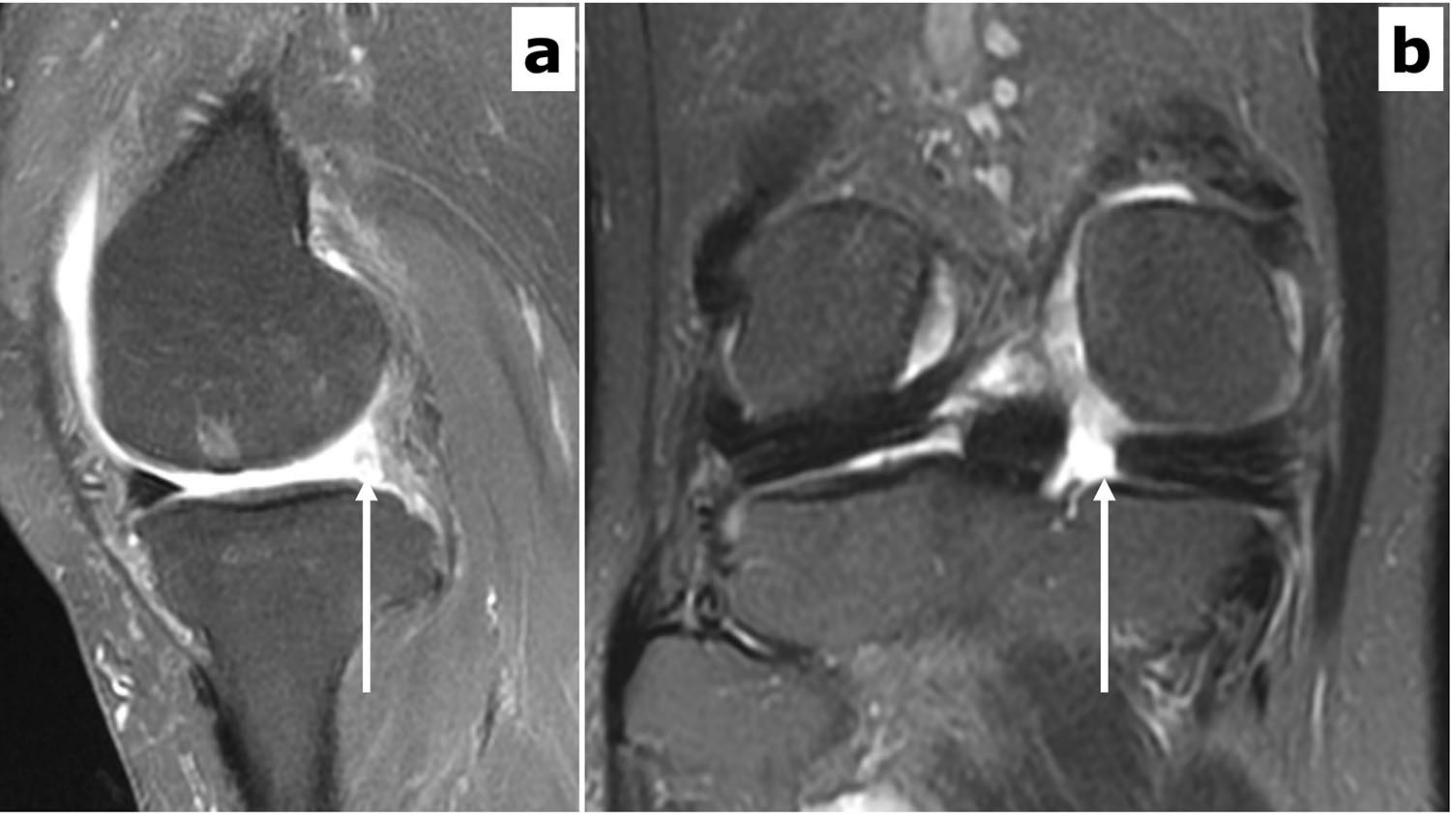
Ghost meniscus sign on a fat-suppressed T2-weighted sagittal image. (a) Sagittal view showing ghost meniscus sign, (b) coronal view showing a defect of the medial meniscus posterior root. An abnormally high signal, indicating the absence of an identifiable meniscal body, is detected in place of the normal dark meniscal signal at the posterior horn of the medial meniscus (arrow).
